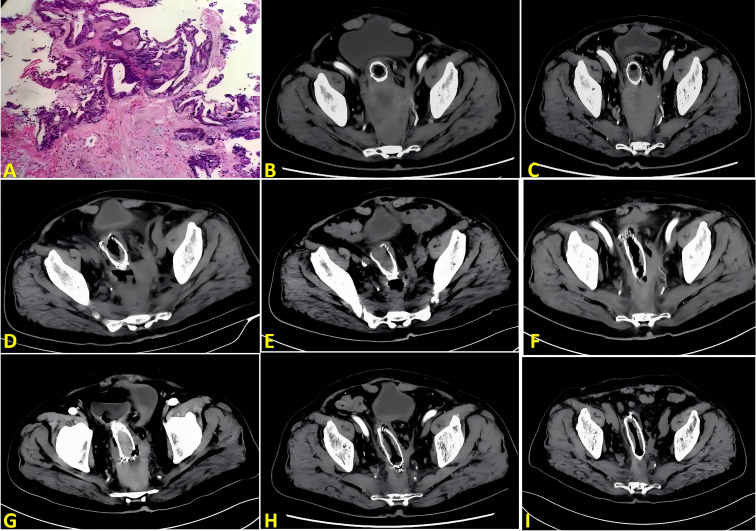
(A) Colonoscopic biopsy pathology revealed moderately to poorly differentiated adenocarcinoma. (B) Contrast-enhanced abdominal CT demonstrated a rectal space-occupying lesion with marked enhancement, measuring 115 mm × 70 mm, invading the bladder with fistula formation, and left hydroureter with hydronephrosis. (C) CT performed 3 weeks after the first interventional procedure showed reduction in tumor size. (D) CT performed 1 week after the third interventional procedure revealed further tumor shrinkage, marked hypodensity, and cavitary necrosis surrounding the stent. (E) Three weeks after the third interventional procedure, the necrotic portion of the tumor was partially absorbed, with further reduction in tumor size and residual necrosis visible internally. (F) Three months after the first interventional procedure, the tumor had markedly decreased in size compared with previous images. (G) Four months after the first interventional procedure, the patient developed recurrent intestinal obstruction; CT showed tumor in growth within the intestinal stent and severe dilatation of the distal colon. (H) One month after palliative radiotherapy, the tumor had significantly regressed and disappeared, with no enhancement observed in residual lesions. (I) One year after the first interventional procedure, CT demonstrated a patent intestinal stent with no evidence of space-occupying lesions or abnormal enhancement at the margins.
